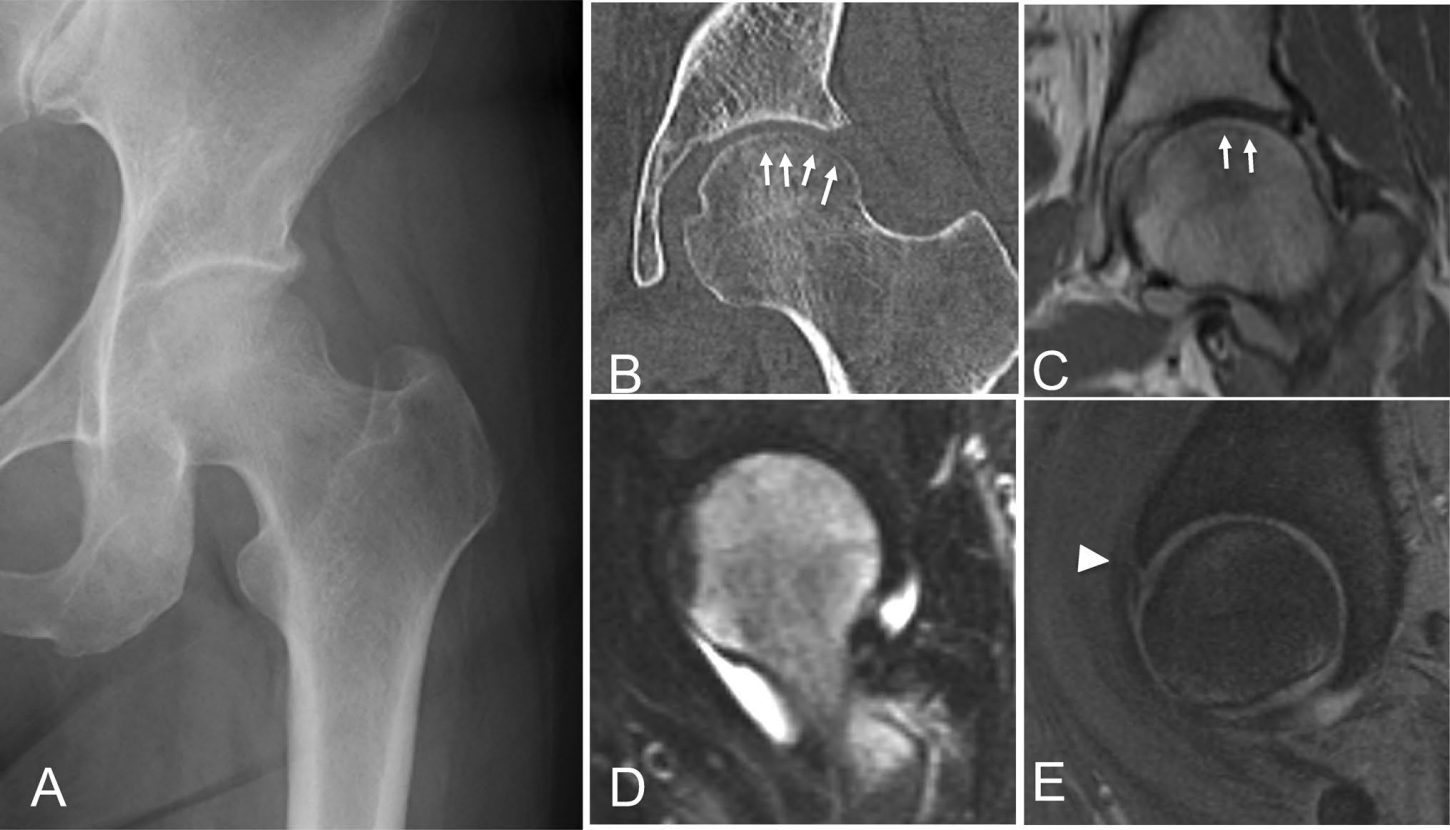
A 45-year-old female presented with 4-month history of increasing left deep groin pain. She had a limited range of motion of 90° of flexion and 15° of internal rotation. She also had a positive anterior and posterior impingement sign. a An anterior–posterior (AP) pelvic view shows LCE angle of 30° and intact Shenton’s line. There was no visible fracture line or loss of sphericity of the femoral head. b Computed tomograph coronal view shows curved hair line paralleled to articular surface of the femoral head. c Proton density coronal views show lo-intensity band parallel to subchondral area along femoral head. d Oblique sagittal MRI short-time inversion recovery (STIR) view shows diffuse high-intensity area suggesting bone marrow oedema pattern in entire lesion of the femoral head. e T2-star-weighted oblique axial view shows anterosuperior labral tear (arrow)
